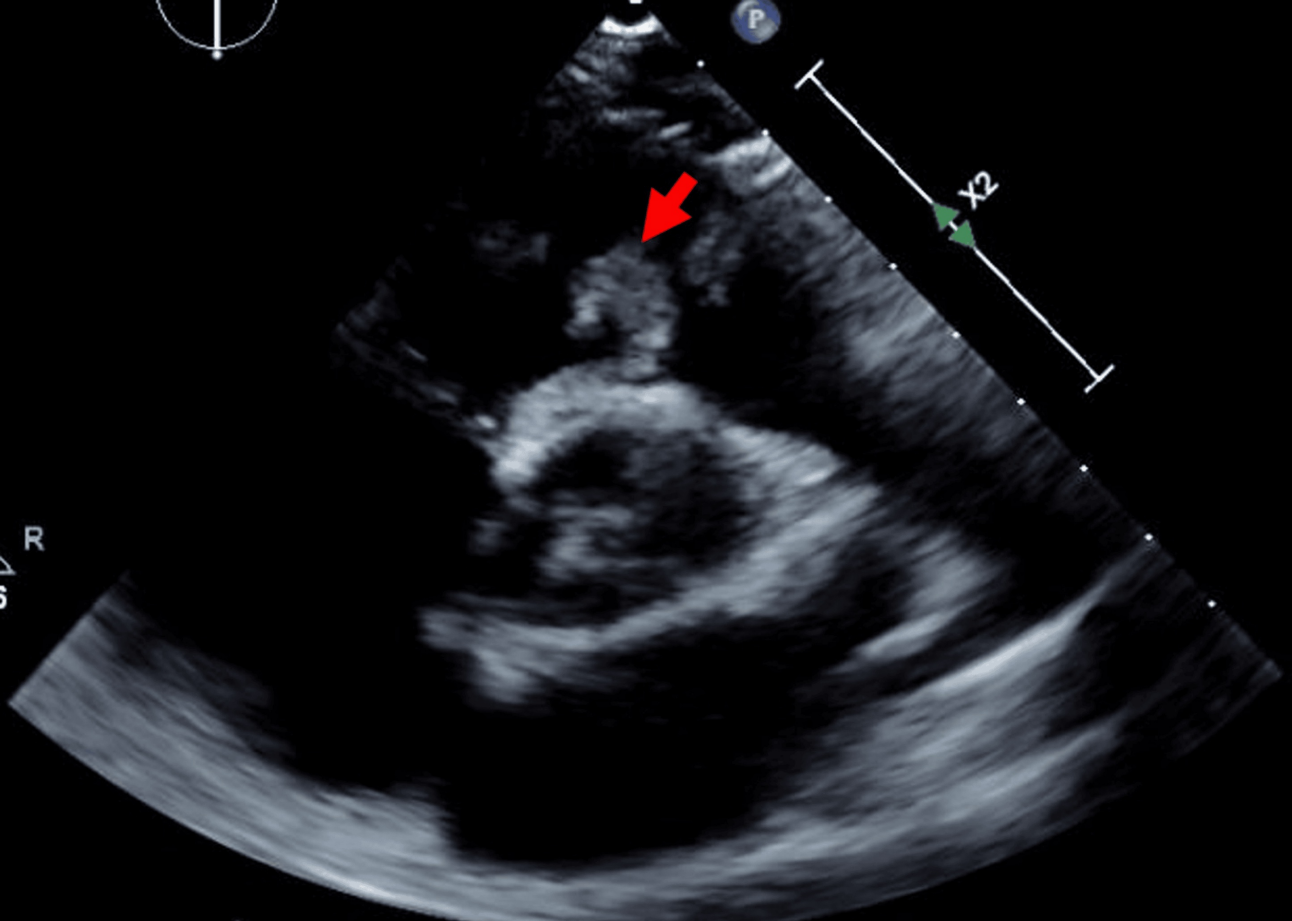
Parasternal short-axis view showing an isolated large irregularly shaped (3.0 cm x 1.5 cm) mass on the pulmonic valve consistent with vegetation. The red arrow depicts the vegetation.
